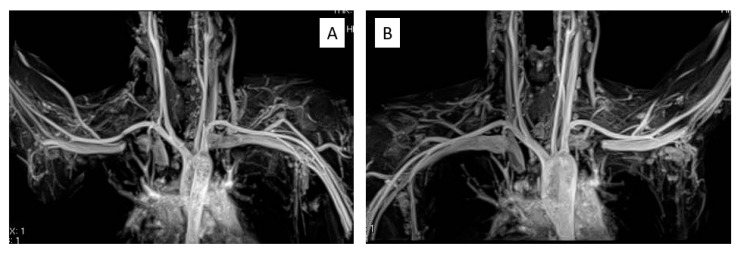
MRI-MIP display from the chest to the upper arm. (A) Raised right arm. (B) Raised left arm. MRI-MIP, magnetic resonance imaging maximum-intensity projection.
